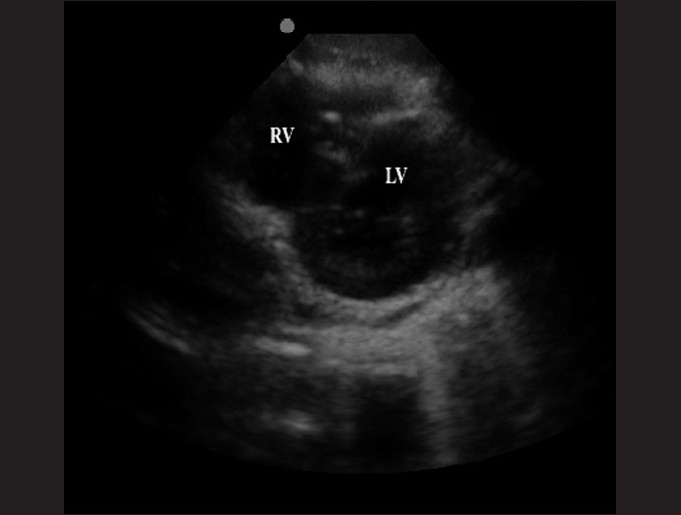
The parasternal short-axis view, also known as the “doughnut” or “fish-mouth” view, of the left ventricle (LV)
